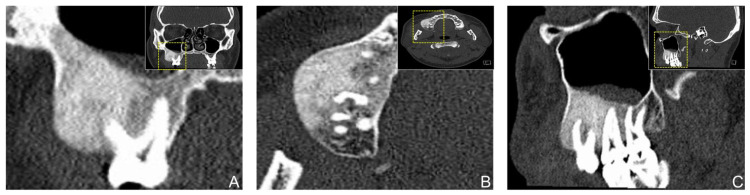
Coronal (A), axial (B), and sagittal (C) reconstruction of a study participant’s computed tomography (CT) showing a fibrous dysplasia in the posterior maxilla. Clinically, the patient presented with swelling associated with tenderness and hypoesthesia.
